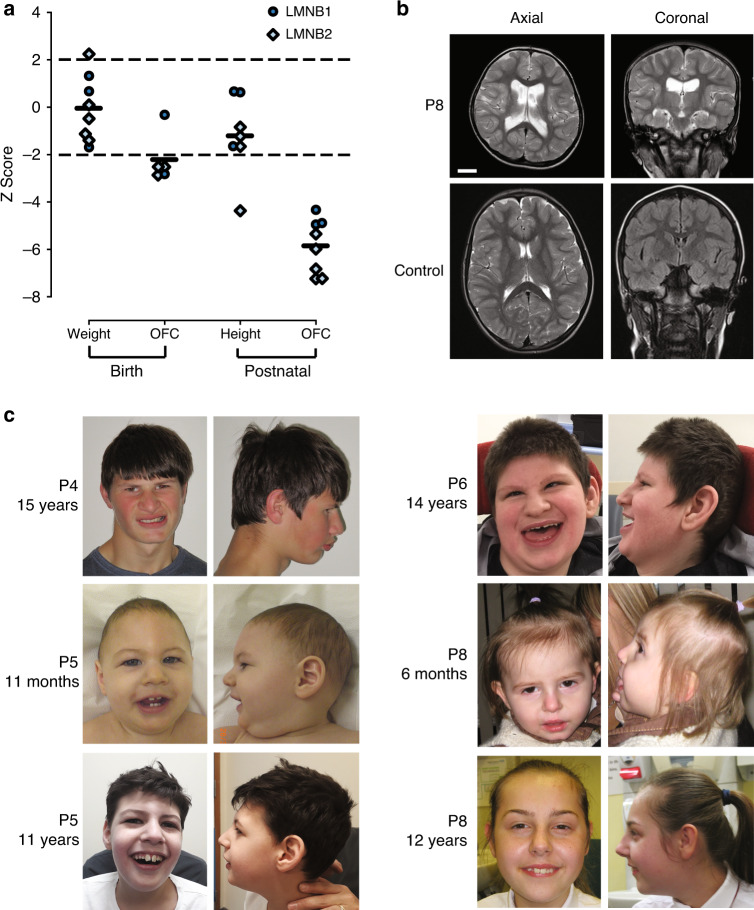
Pathogenic *LMNB1* or *LMNB2* variants in patients with primary microcephaly. (a) Growth parameters of individuals with pathogenic *LMNB1/2* variants plotted as *Z*-scores (standard deviations from the age and sex-matched mean). Black bars, mean *Z*-score. Dashed lines, 95% confidence interval for general population. (b) Neuroimaging demonstrates reduced cortical size with simplified gyri. Ventricular dilatation reflects accompanying global reduction in white matter. Comparison with age-matched control. Scale bar 2 cm. Axial images, T2-weighted. Coronal, T2 (P8), fluid-attenuated inversion recovery (FLAIR) (control). (c) Photographs of individuals P4, P5, P6, and P8. Written consent obtained from families for photography. *OFC* occipital frontal circumference.
